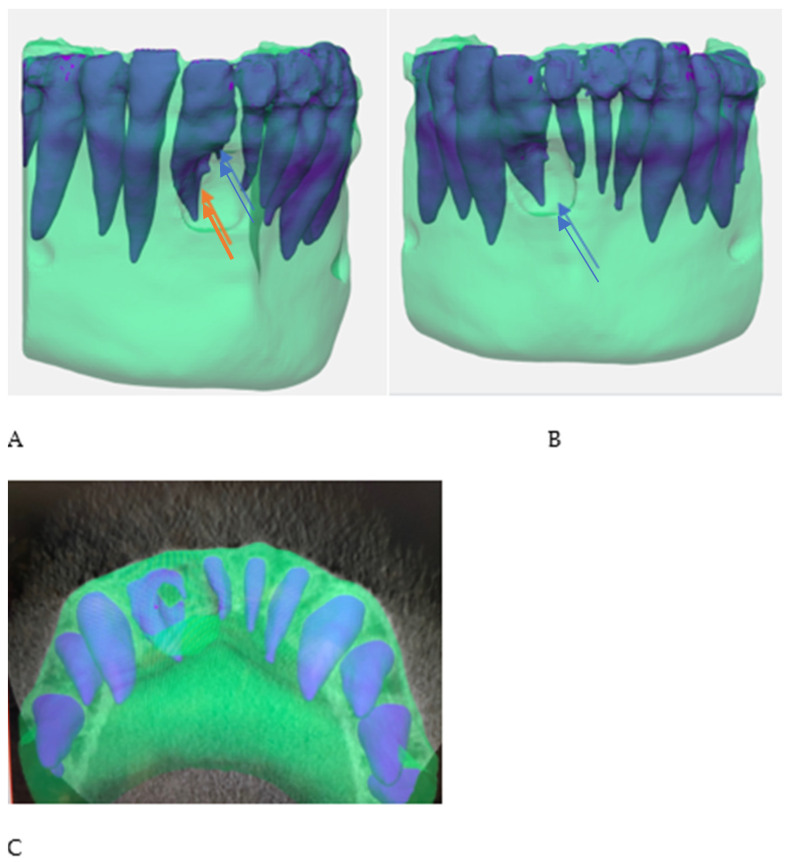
3D jaw models utilized in pre-surgical planning. 3D model showing the mesiofacial projection: (A) (blue arrow) the lingual wing; (A) (orange arrow) apical aspect of the DI with lingual wing formation; (B) extent of periapical bony lesion; and (C) axial view representation.
